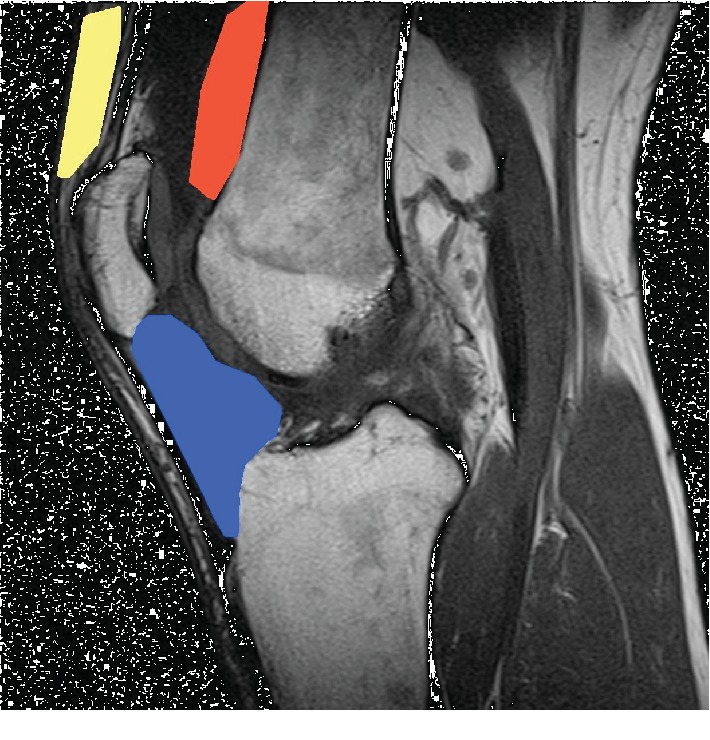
Sagittal knee MRI with periarticular fat sources (yellow—subcutaneous fat; red—prefemoral/supratrochlear pouch fat; blue—infrapatellar fat pad/Hoffa's fat pad).
